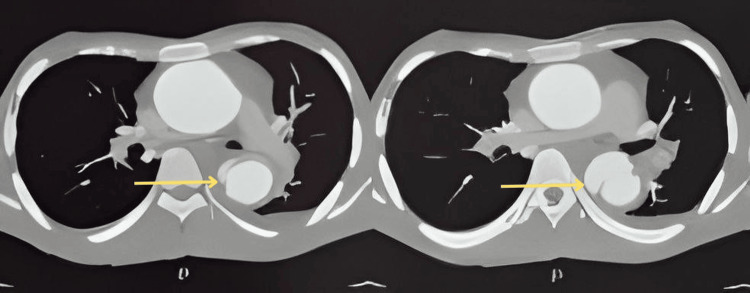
Axial chest computed tomography at the T7 and T8 level demonstrating aortic dissection in the descending aorta with an intimal flap.
